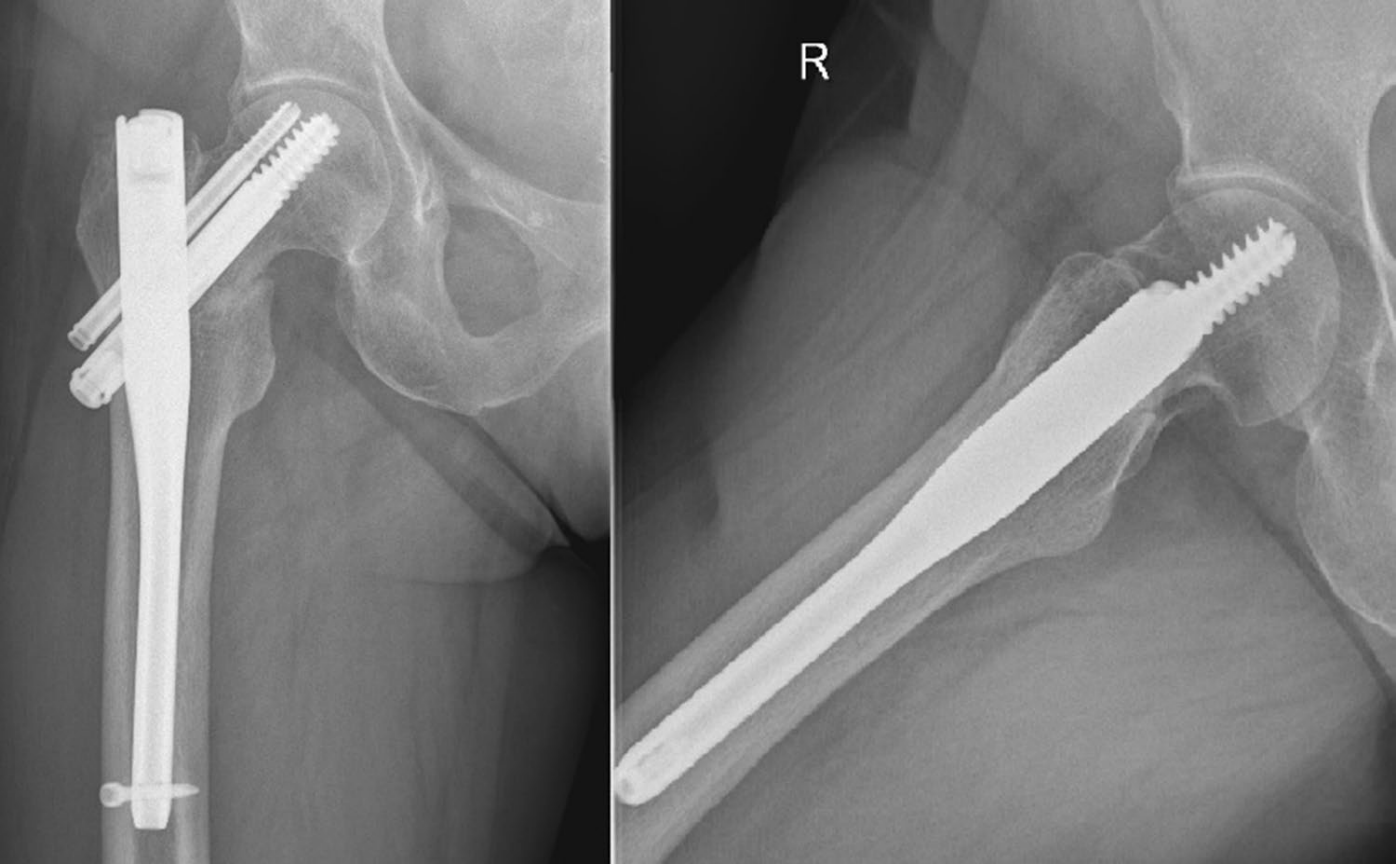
The anteroposterior and axial radiograph 3 months after the surgery
